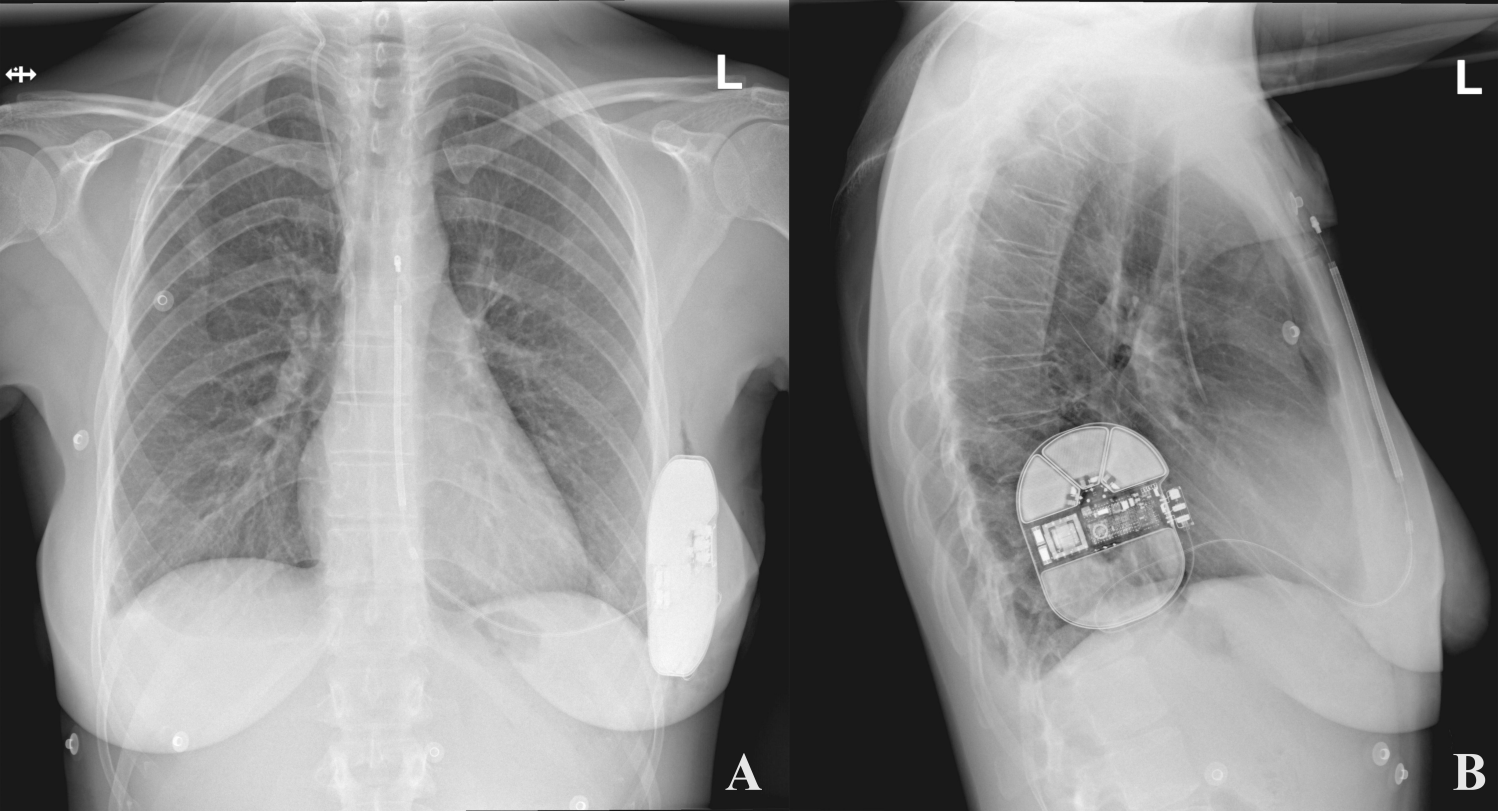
An exemplary postoperative chest x-ray presenting an appropriate
